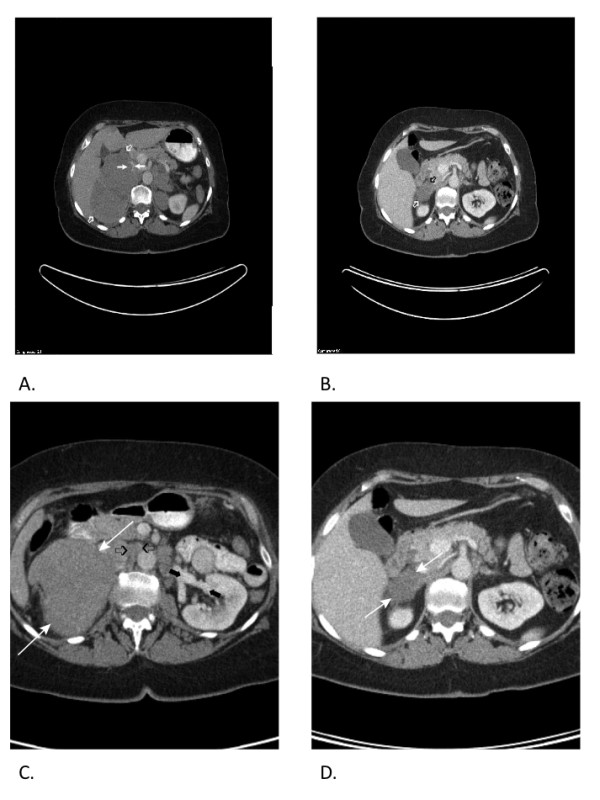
Right renal mass and inferior vena cava (IVC) thrombus. Renal mass and IVC thrombus. (A) Computed tomographic (CT) image showing large renal mass (open white arrows) invading right adrenal gland, liver and IVC. Note intravascular thrombus in the IVC (solid white arrows). (B) CT image showing marked improvement in renal mass after three cycles of cyclophosphamide, doxorubicin, vincristine, prednisone plus rituxumab (R-CHOP) chemotherapy (open arrows). (C) CT image showing normal left renal vein (black solid arrow), but the right renal vein is not seen (black open arrows), consistent with occlusion by malignant thrombus. White arrows identify the renal tumor. (D) CT image shows improvement of right renal mass.
